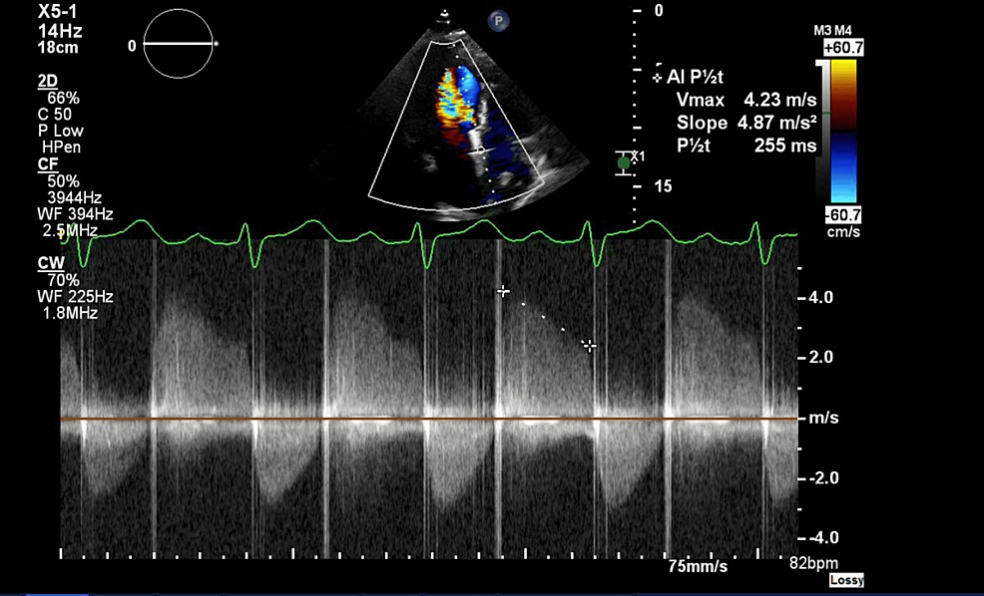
Spectral Doppler showing moderate to severe paravalvular aortic regurgitation
